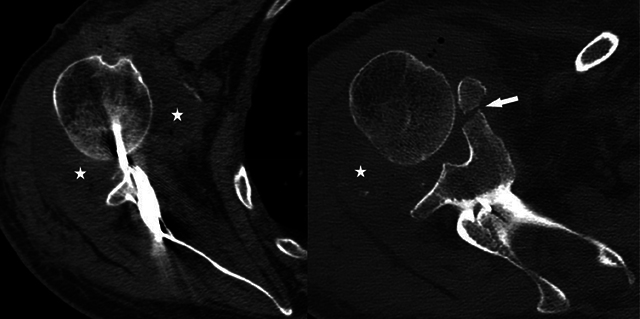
Postoperative axial CT scans of the right shoulder showing displaced coracoid process fracture (arrow), glenohumeral joint effusion (asterisks), and anterior humeral head subluxation.
